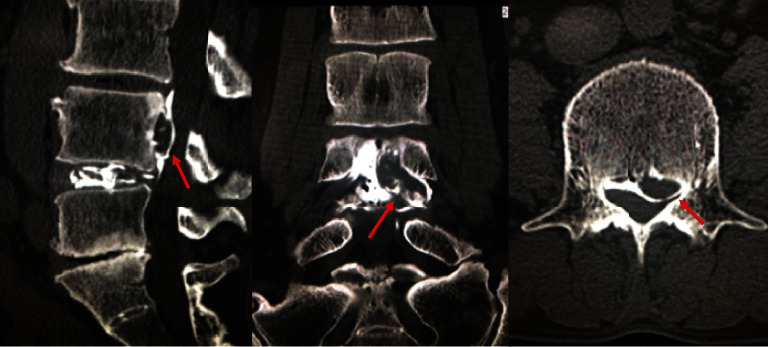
The herniated fragment was migrated from neural foramen to cranial central canal on discography-enhanced CT.
